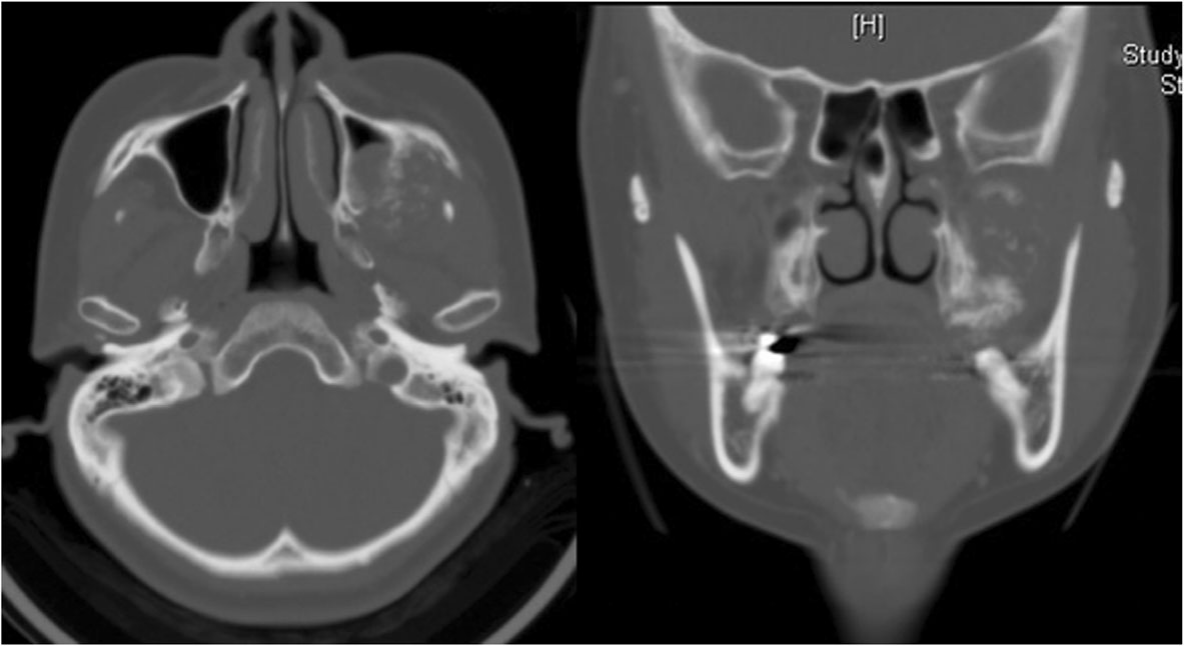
Computed tomography image showing heterogeneous bone mass. Feasibility of medullary bone invasion toward the inferior orbital fissure via pterygopalatine fossa
